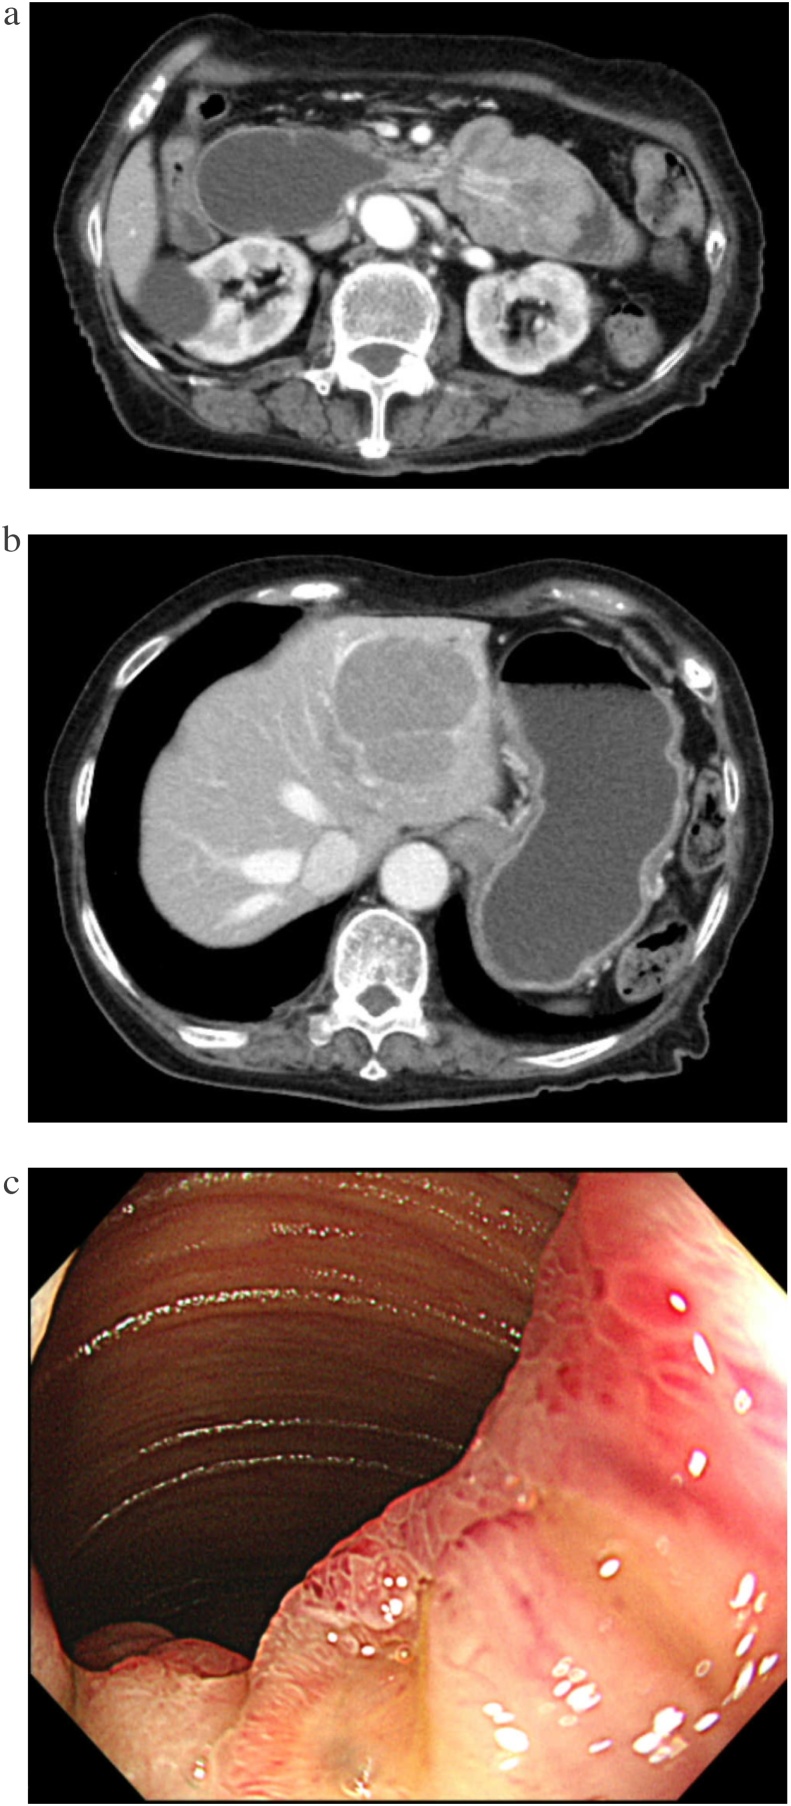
a) Findings on contrast-enhanced computed tomography. Intussusception of the third portion of the duodenum is seen, as is duodenal dilation on the oral side of the lesion in the arterial phase. b) Findings on computed tomography in the equilibrium phase. Two masses without exophytic growth in the left hepatic lobe are seen, suggesting metastasis. c) Findings on esophagogastroduodenoscopy. A protruding lesion in the third portion of the duodenum is seen, which the scope is able to pass through.
